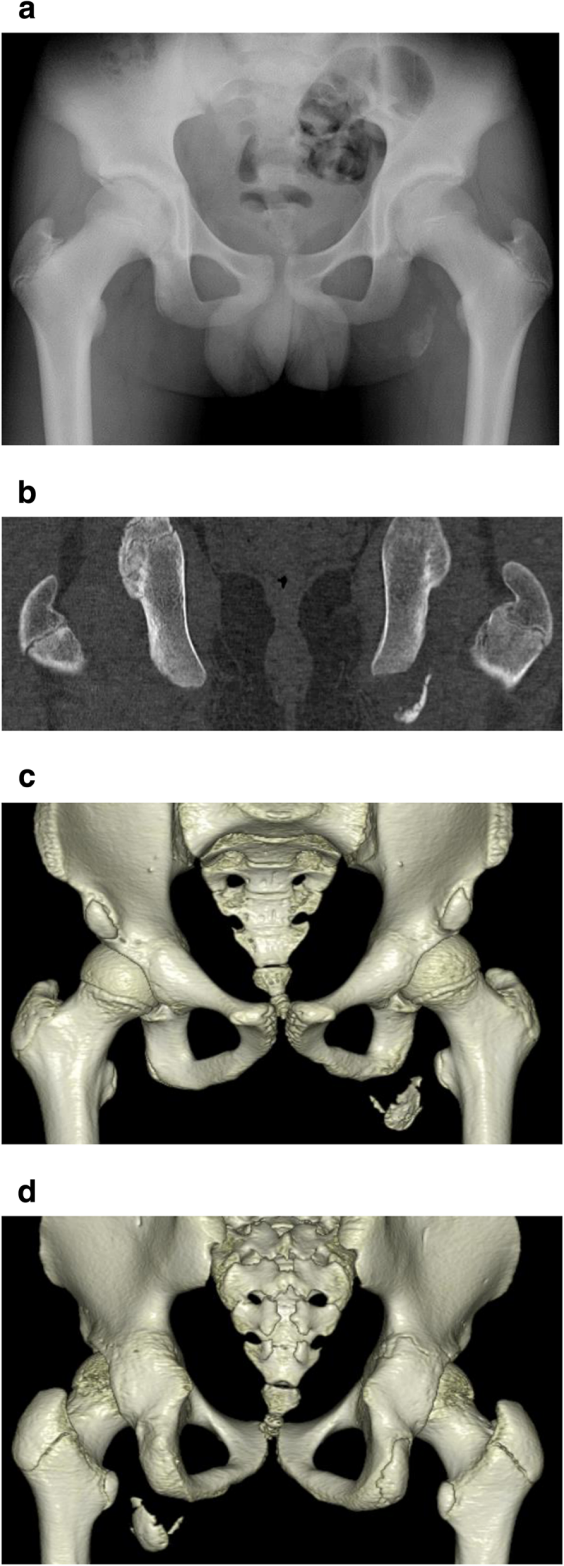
a Preoperative radiography reveals left ischial epiphyseal fracture. b CT shows 20 mm of displacement. c Anteroposterior preoperative three-dimensional CT (3D-CT). d Posteroanterior preoperative 3D-CT
